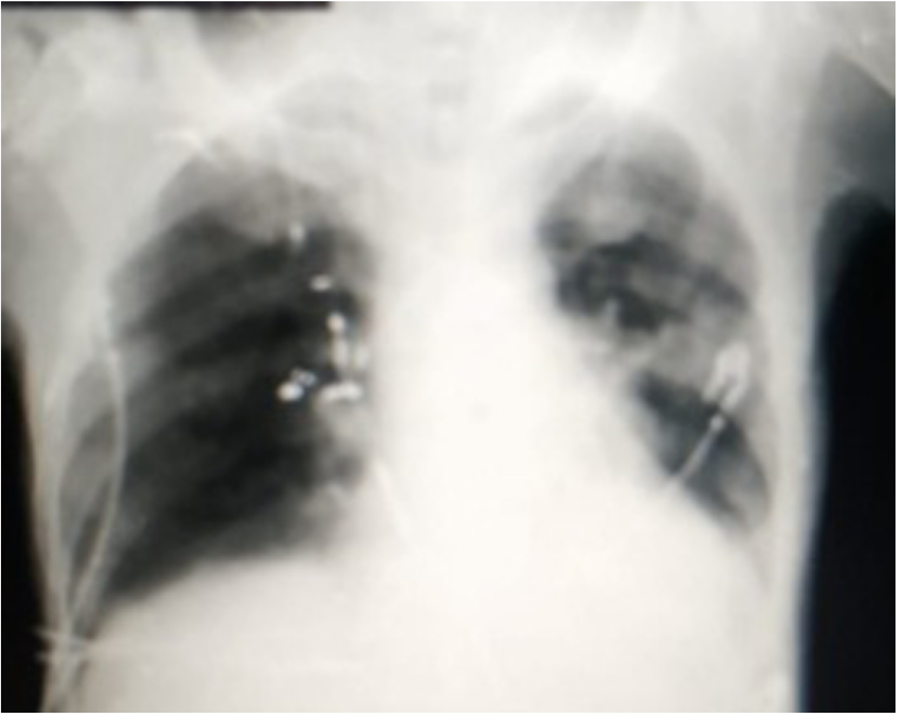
Chest X-ray (day 1) revealing bilateral ground-glass opacities more at the left lung
